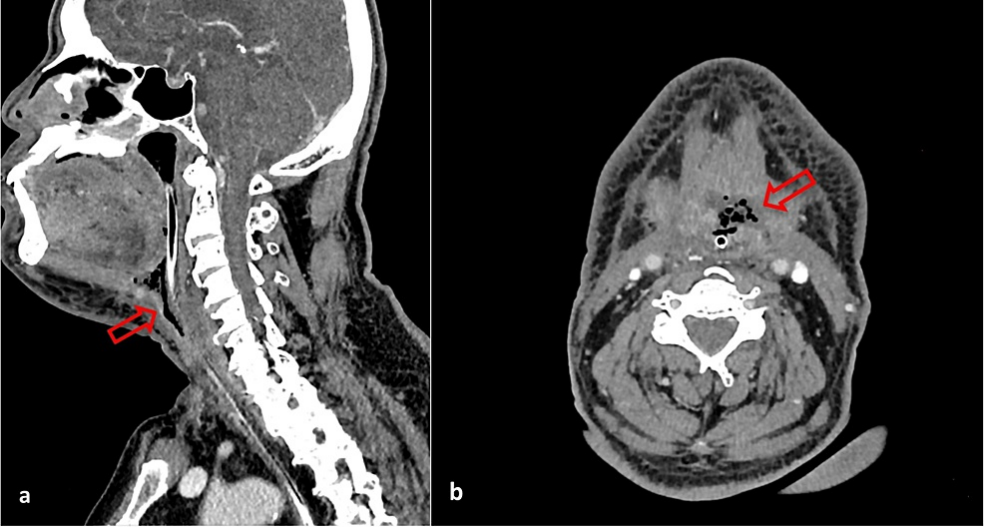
The red arrows in the (a) sagittal and (b) axial CT scan images show multiloculated, rim-enhancing collection anterior to the neopharynx, extending to the submental region at the C3/C4 level and measuring approximately 1.5 cm × 3.3 cm × 1.5 cm.
